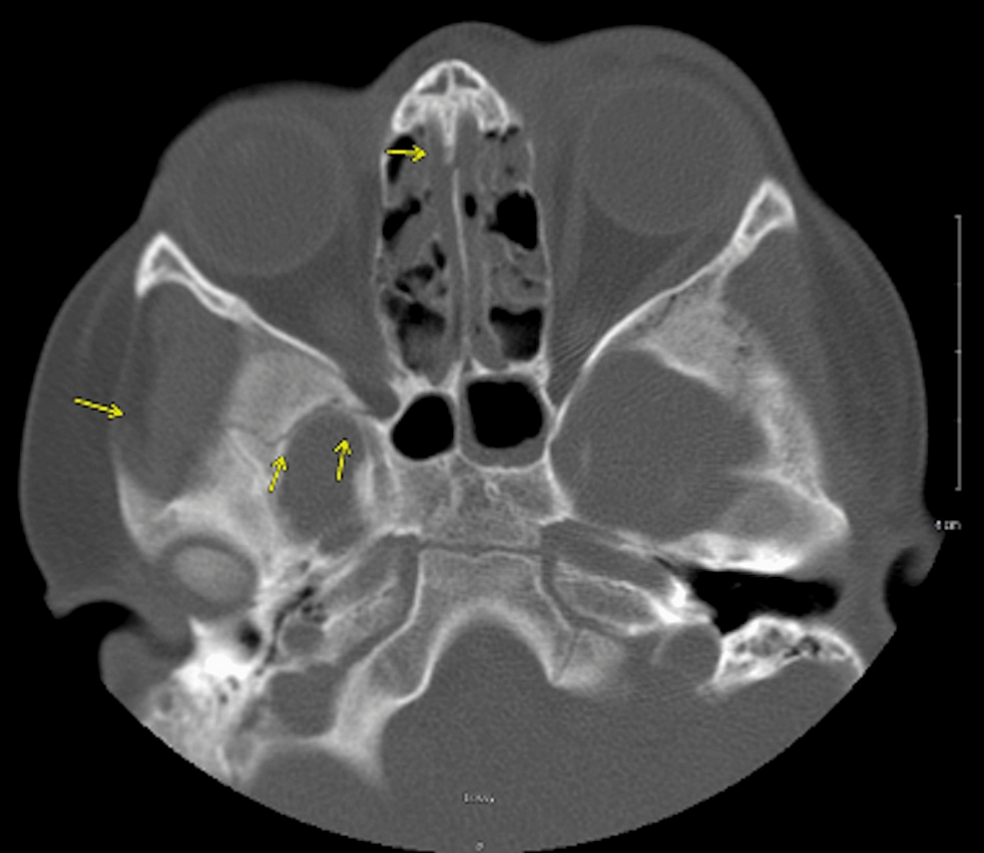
CT scan of the head CT scan of the head from six months prior when the patient was injured causing facial fractures. The following findings from the CT scan: left nasal bone fracture, bilateral impacted medial orbital wall fractures with one posteriorly on the right with the most prominent fracture, minimally displaced fracture of the right inferior temporal fossa, minimally displaced sphenoid bone, fracture of anterior cranial fossa at the cribriform plate, and oblique fracture of the left supraorbital frontal bone.
